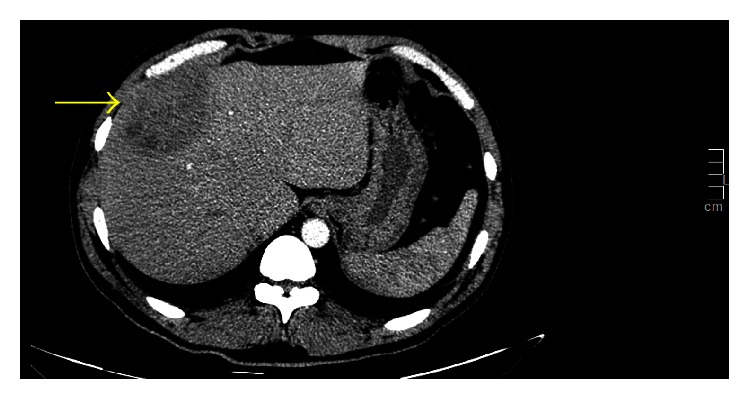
Whole abdominal triphasic CT scan showing an ill-defined, solid-cystic focus exhibiting peripheral enhancement in segment 8 of the liver measuring 7.5 × 6.8 × 6.1 cm.
